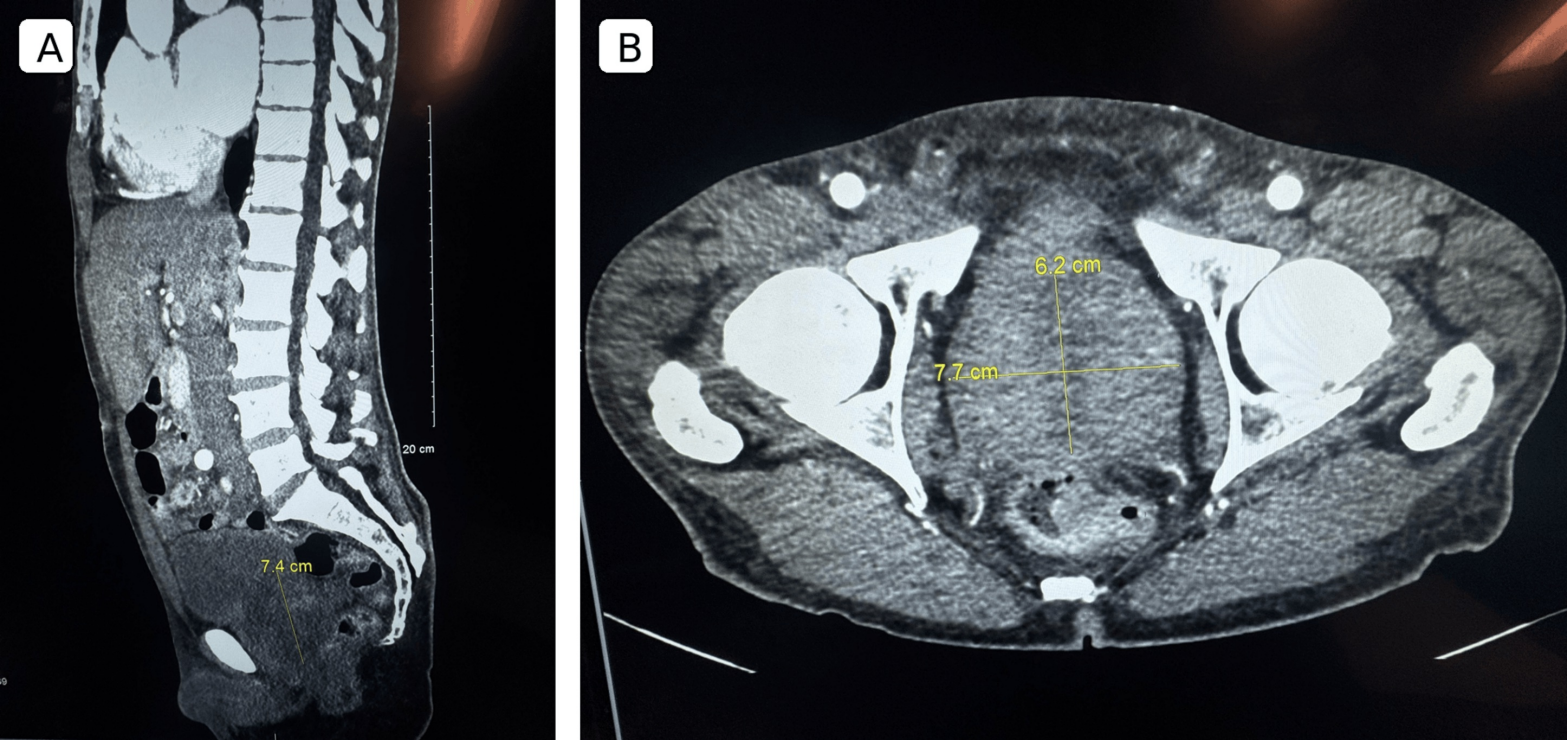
Computed tomography of the abdomen and pelvis obtained during the same admission demonstrating a markedly enlarged, heterogeneous prostate measuring 7.4×6.2×7.7 cm, indenting the bladder base
